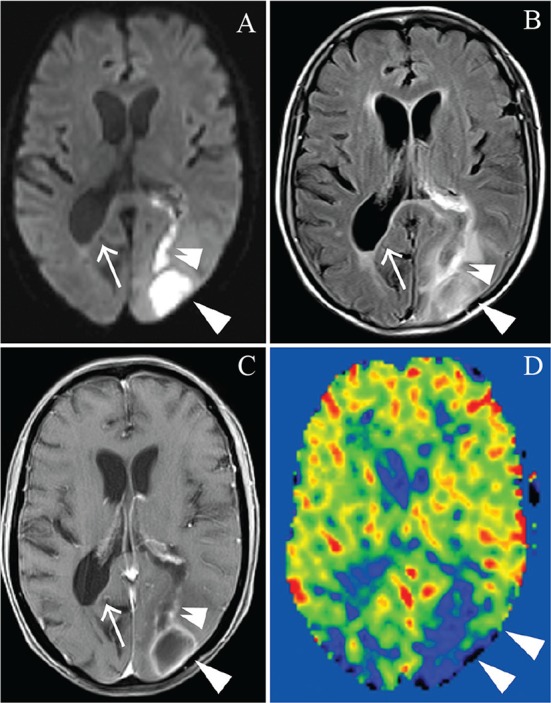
An 80-year-old woman with abscess formation and ventricular empyema due to unspecified bacterial infection. Diffusion weighted image and fluid-attenuated inversion recovery depict the abscess formation (A, B: arrowheads) with intraventricular penetration (A, B: double arrowheads). In addition, these images delineate the development of ventricular inflammation as a high intensity area along the posterior wall of the right lateral ventricle (A, B: arrows). Postcontrast T_1_WI (weighted image) shows ring-like enhancement around the abscess (C: arrowheads) as well as enhancement along the perforating path (C: arrow) and right posterior periventricular wall (C: double arrowheads). Arterial spin-labeling magnetic resonance imaging shows low perfusion only around the abscess areas (D: arrowheads), and reveals no abnormal perfusion in the ventricular wall.
